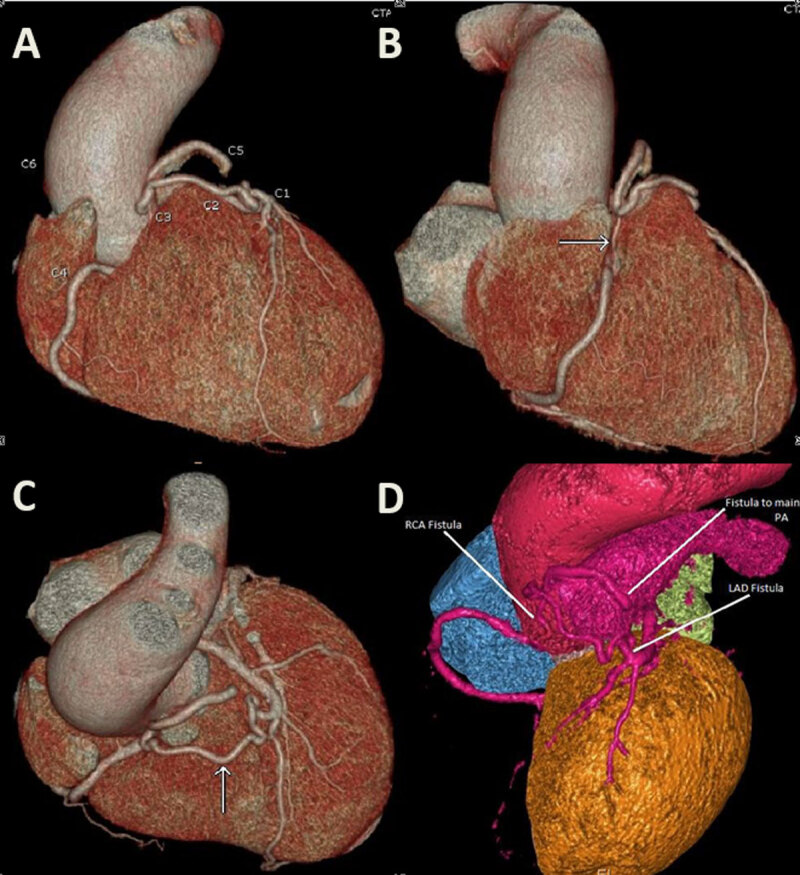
A coronary computed tomography angiography 3-dimensional (3D) reconstruction showing a coronary artery fistula arising from the left anterior descending artery joining another fistula arising from the right coronary artery, both draining into the main pulmonary artery. (A) C1: left anterior descending artery; C2: left anterior descending artery fistula; C3: right coronary artery fistula; C4: right coronary artery; C5: drainage into the pulmonary artery; C6: ectasia of the ascending aorta; (B) arrow: fistula connecting to the right coronary artery; (C) arrow: fistula connecting to the left anterior descending artery; (D) 3D reconstruction of the fistula excluding the right ventricle.
